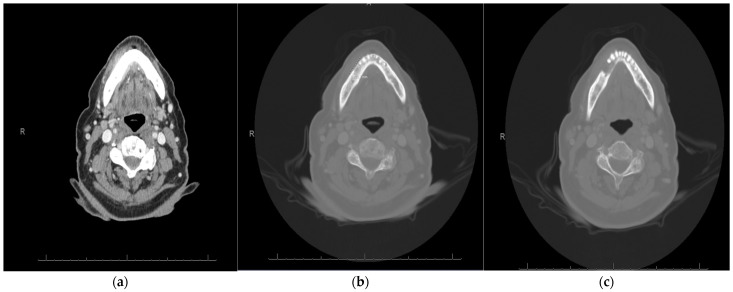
Pretreatment radiologic evaluation of a (a) soft tissue and (b) bone window of an axial slice computed tomography scan showing a 2 cm area of right buccal soft tissue thickening consistent with known tumor. In a subsequent (c) bone window, axial view, osteolysis and marrow invasion is noted adjacent to known carcinoma.
